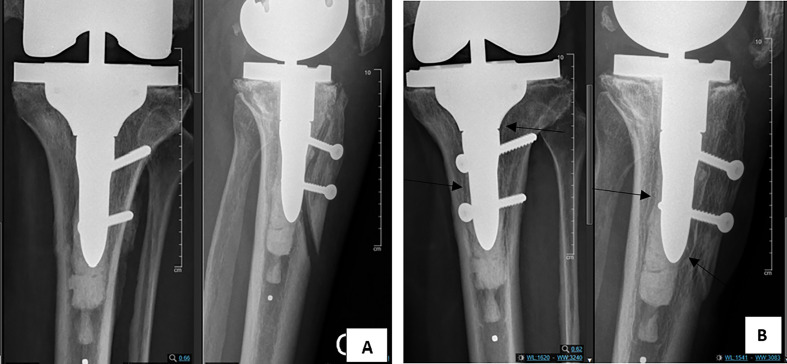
(A) Anteroposterior and lateral post operative radiographs of tibial short stem. (B) Anteroposterior and lateral radiographs of tibial short stem showing radiolucent lines (arrows) and loosening at 3 years follow-up.
